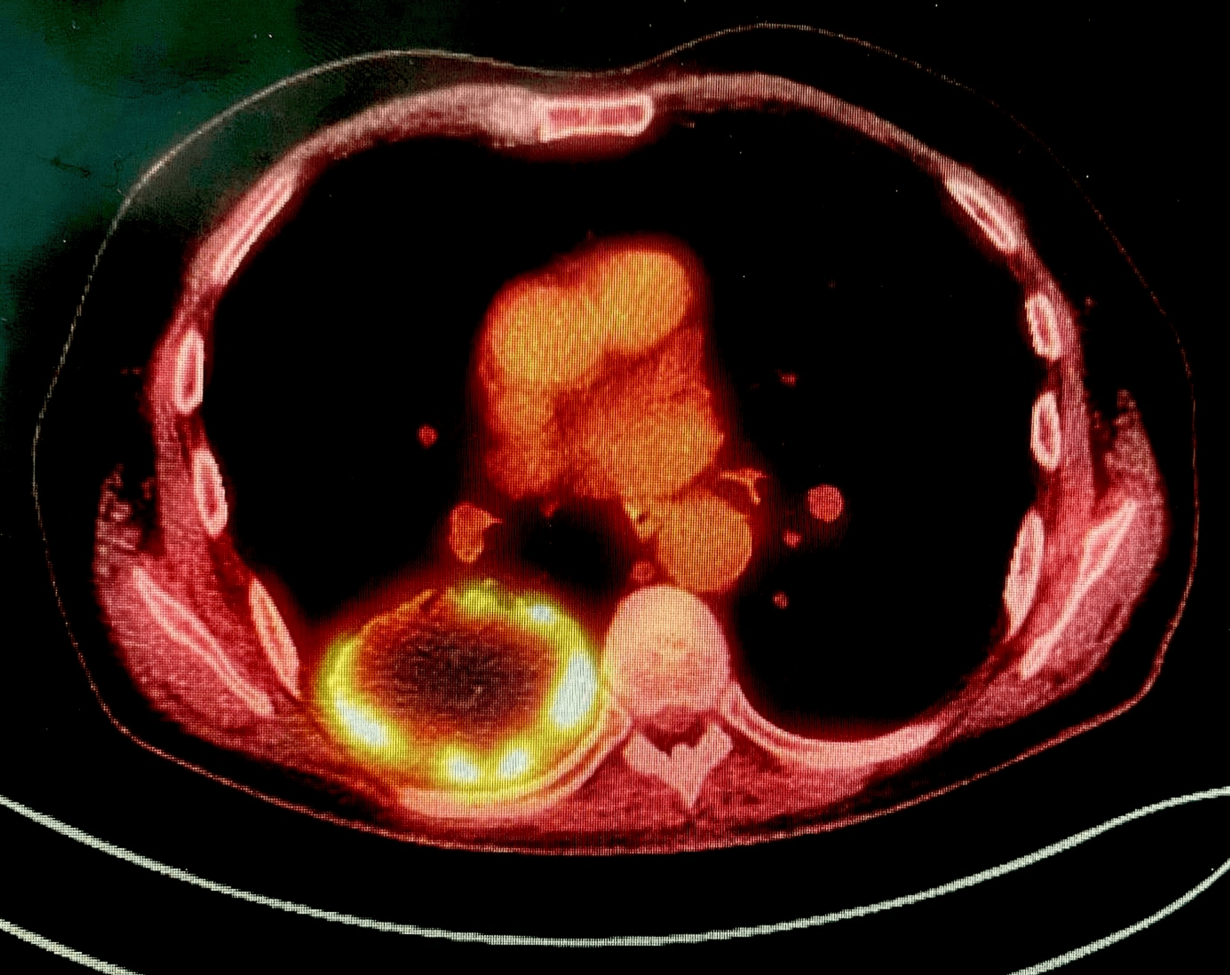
PET-CT chest shows a PET-avid mass. PET-CT, positron emission tomography-computed tomography
